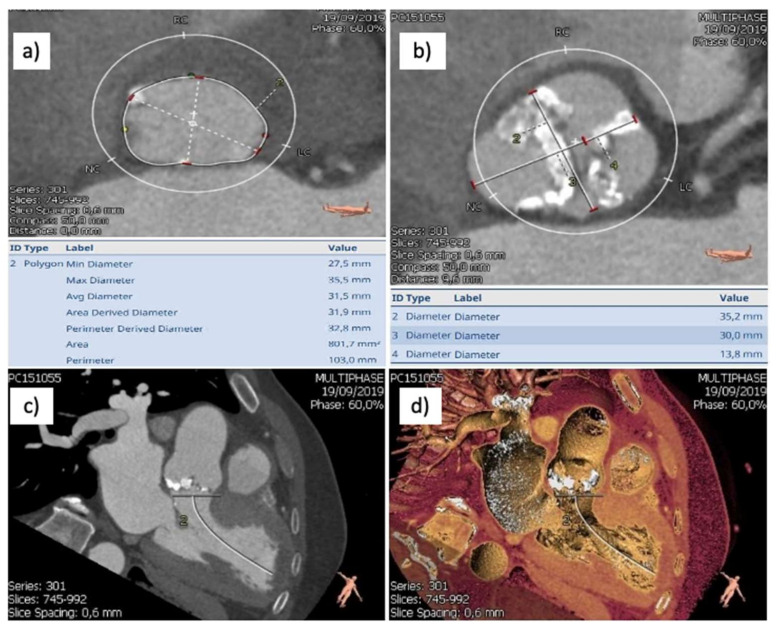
(a) Aortic annulus aspect on angio CT-large aortic annulus; (b) aortic valve aspect on angio CT-type 1 Sievers, left to right cusp fusion, severe asymmetric calcifications; (c,d) MDCT image showing significant NCS calcifications.
